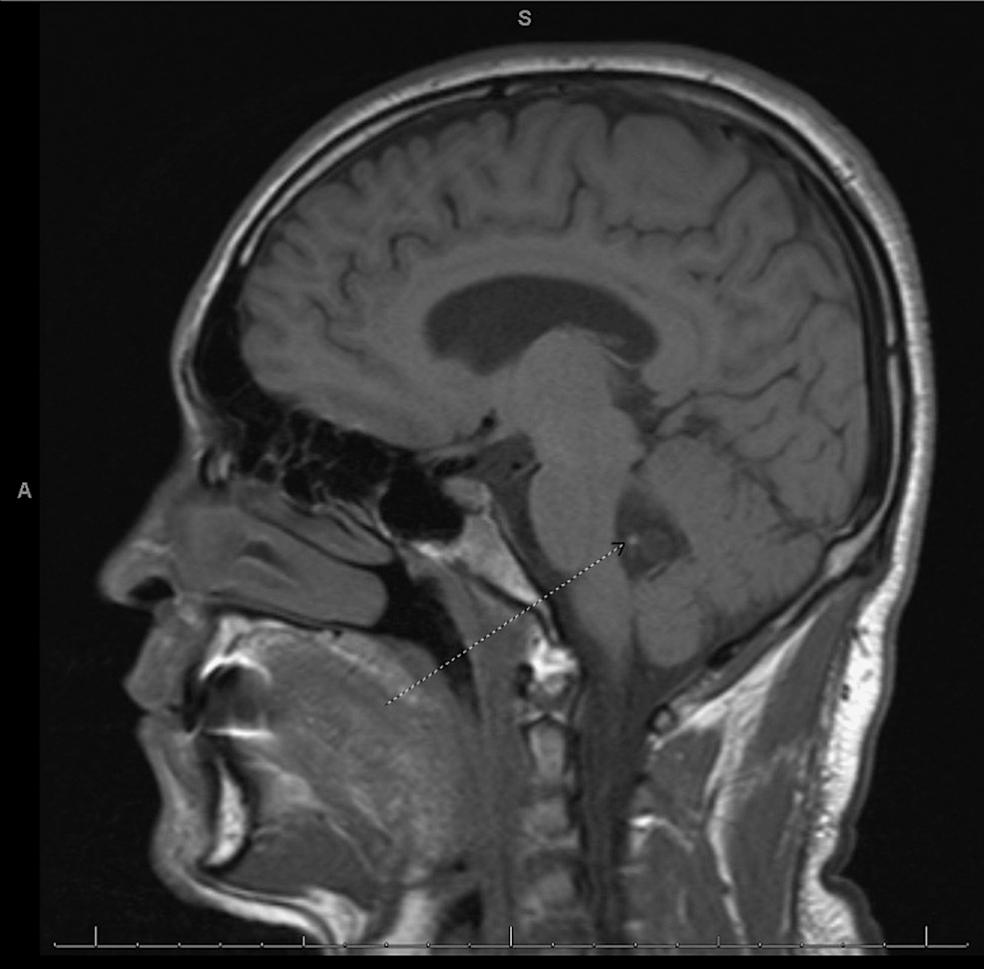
Brain MRI sagittal plane shows a cystic-appearing lesion in the fourth ventricle (arrow).
